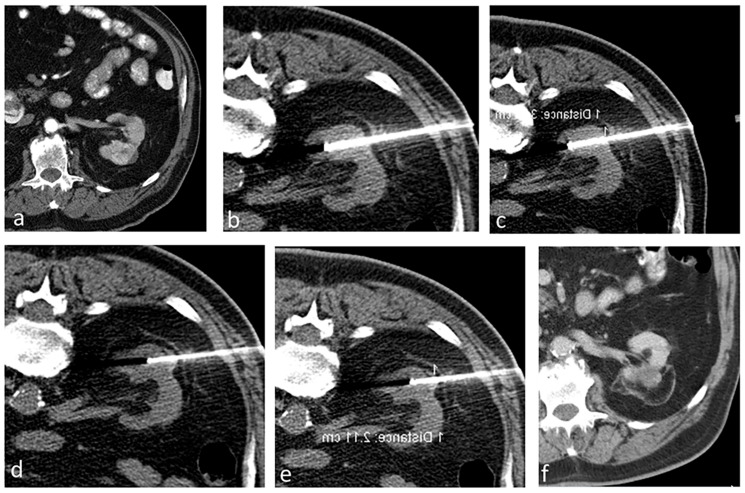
A patient with a 3.9 cm biopsy-proven RCC treated using RFA. (a) Contrast-enhanced CT showing a renal mass (3.9 cm in transverse diameter) in the left kidney. (b–e) Ablation procedure. (b,c) Non-contrast axial CT showing the probes positioned to treat the deeper portion of the tumor, and subsequently the probes were retracted to treat the superficial portion of the tumor (d,e). (f) Contrast-enhanced CT after 5 years showing no evidence of residual or recurrent tumor in the left kidney.
